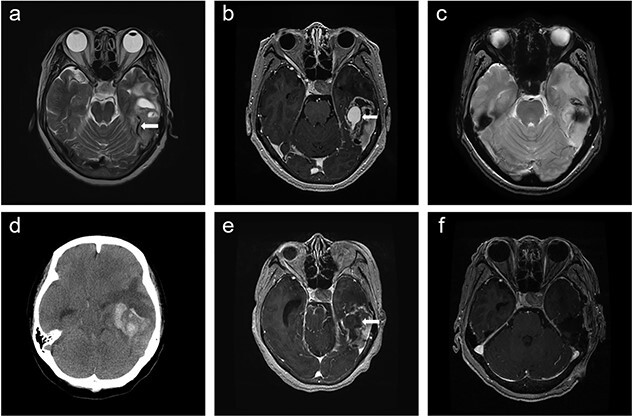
Time course of brain images during intratumoral hemorrhage formation; (a) initial T2-weighted image showing the tumor-draining vein without venous ectasia (arrow); (b, c) T1-enhanced image and gradient-echo image obtained 1 week after the initial MRI showing new-onset venous ectasia (arrow) without internal thrombus; (d, e) non-contrast-enhanced brain CT and T1-enhanced images obtained 1 day after images; (b) and (c) showing intratumoral hemorrhage with an intra-luminal thrombus (arrow); (f) post-operative T1-enhanced image.
